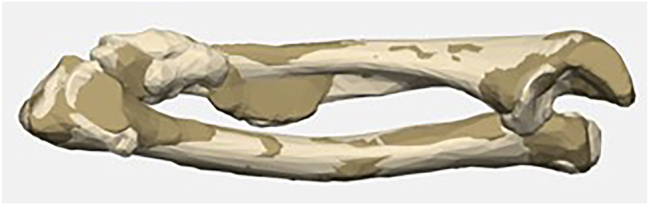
Case 5: Comparison of CT-generated model (light) and MRI-based model (dark).
